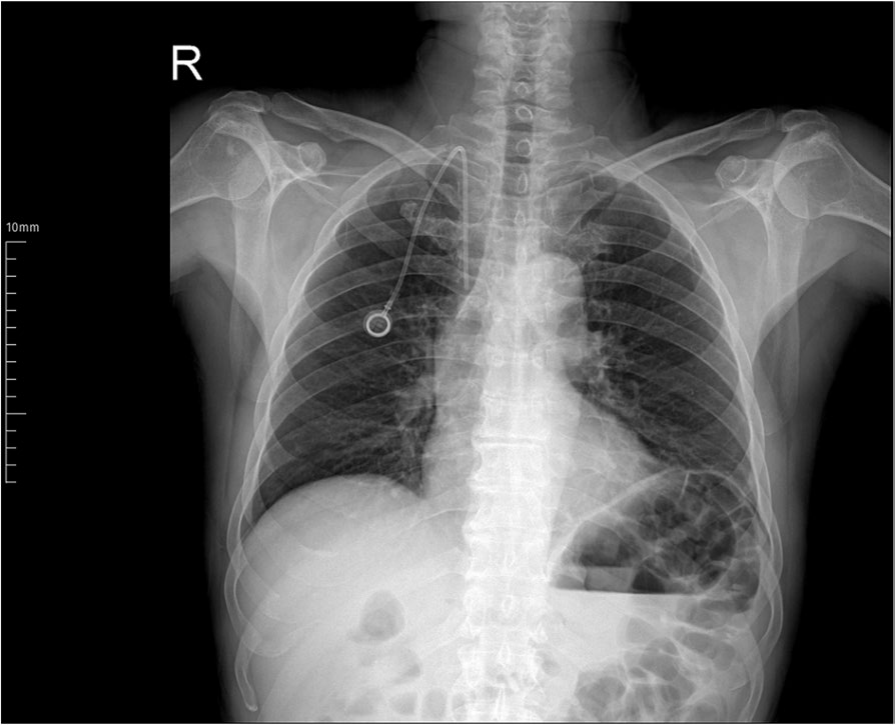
A chest radiograph obtained three months after the catheter was repositioned revealed that the catheter tip remained in the same location
